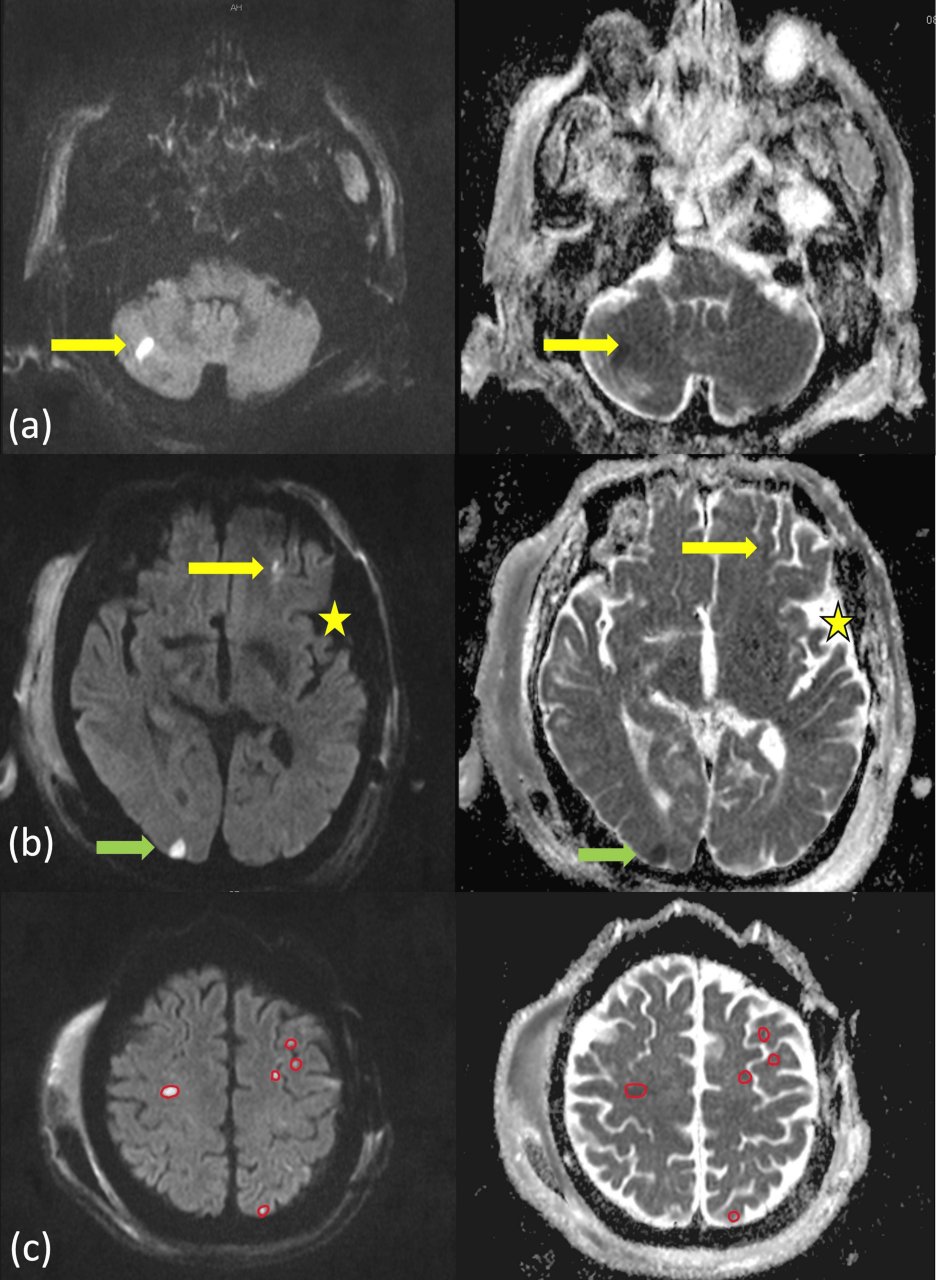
MRI findings on DWI and ADC sequences Axial cuts from DWI (left panel) and ADC (right panel) sequences demonstrate (a) hyperintensities on DWI with corresponding hypointense and some isointense areas on ADC in the cerebellar region (yellow arrows), (b) punctate foci of diffusion restriction at the level of the third ventricle, involving the left frontal territory (yellow arrow) and right occipital lobe (green arrow), along with evidence of a prior left craniotomy (yellow star), and (c) multiple areas of diffusion restriction at the level of the centrum semiovale, involving frontoparietal distributions. These findings indicate acute to subacute strokes in both anterior and posterior circulations, raising concern for a possible cardiogenic source of emboli. ADC, apparent diffusion coefficient; DWI, diffusion-weighted imaging
